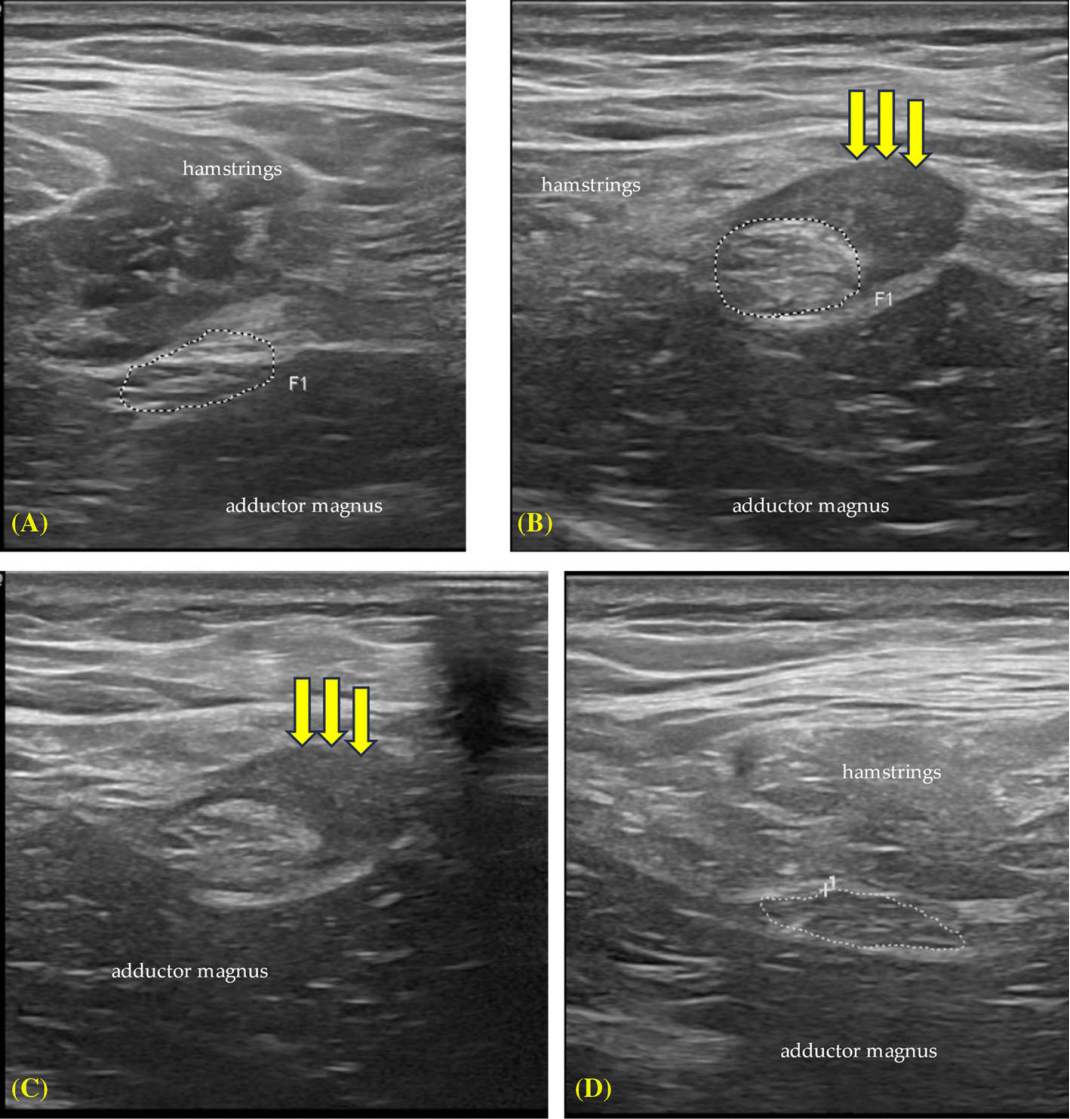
Ultrasound images. (A) Asymptomatic right sciatic nerve in the posterior thigh 9 cm distal to the ischial tuberosity (IT). (B) Left sciatic nerve in the posterior thigh 9 cm distal to the IT. Nerve is enlarged. Hamstrings are retracted with hyperechogenicity and loss of muscle echotexture. Hypoechoic structure (yellow arrows) consistent with developing scar tissue tightly tethers sciatic nerve to retracted hamstrings. (C) Left sciatic nerve in the posterior thigh 16 cm distal to the IT. Again, nerve is enlarged with tethering to retracted hamstrings. Nerve was found to be tethered to hamstrings from IT to 18 cm distally. (D) Left sciatic nerve in the posterior thigh 20 cm distal to IT. Normal appearance.
